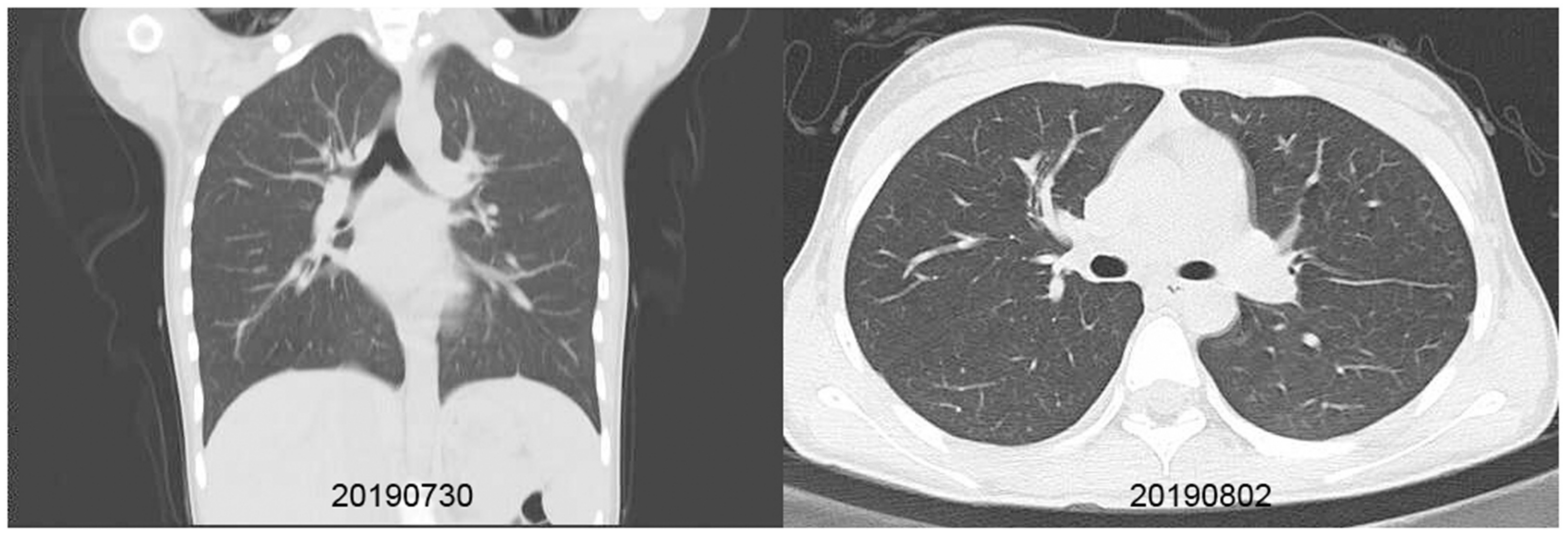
Chest X-ray and CT scan taken for a 13-year-old girl with HCoV-229E and adenovirus coinfection-induced fever on July 27, 2019. Chest CT scan and chest X-ray indicated slight thickening of the wall of the anterior and posterior segments of the right upper lobe, a slightly narrower lumen, and slight inflammation of the lower right lung. The bilateral hilum was normal, and no pleural effusion was observed. No obvious swollen lymph node shadow was found in the mediastinum. (A) Left, Chest X-ray taken on July 30, 2019. (B) Right, Chest CT scan taken on August 2, 2019.
